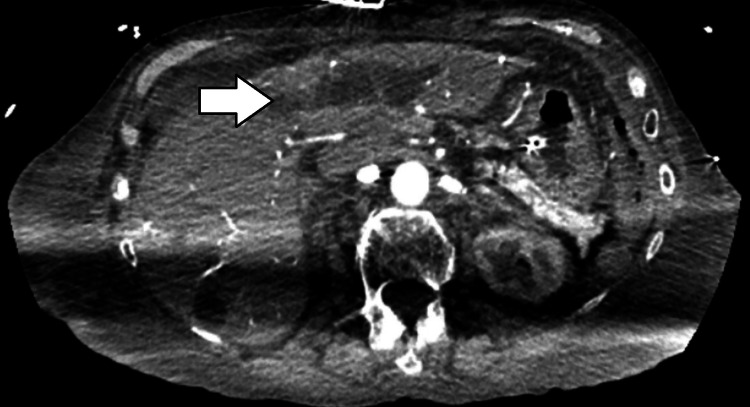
Computed tomography of the abdomen showing left biliary ductal dilation (white arrow)
